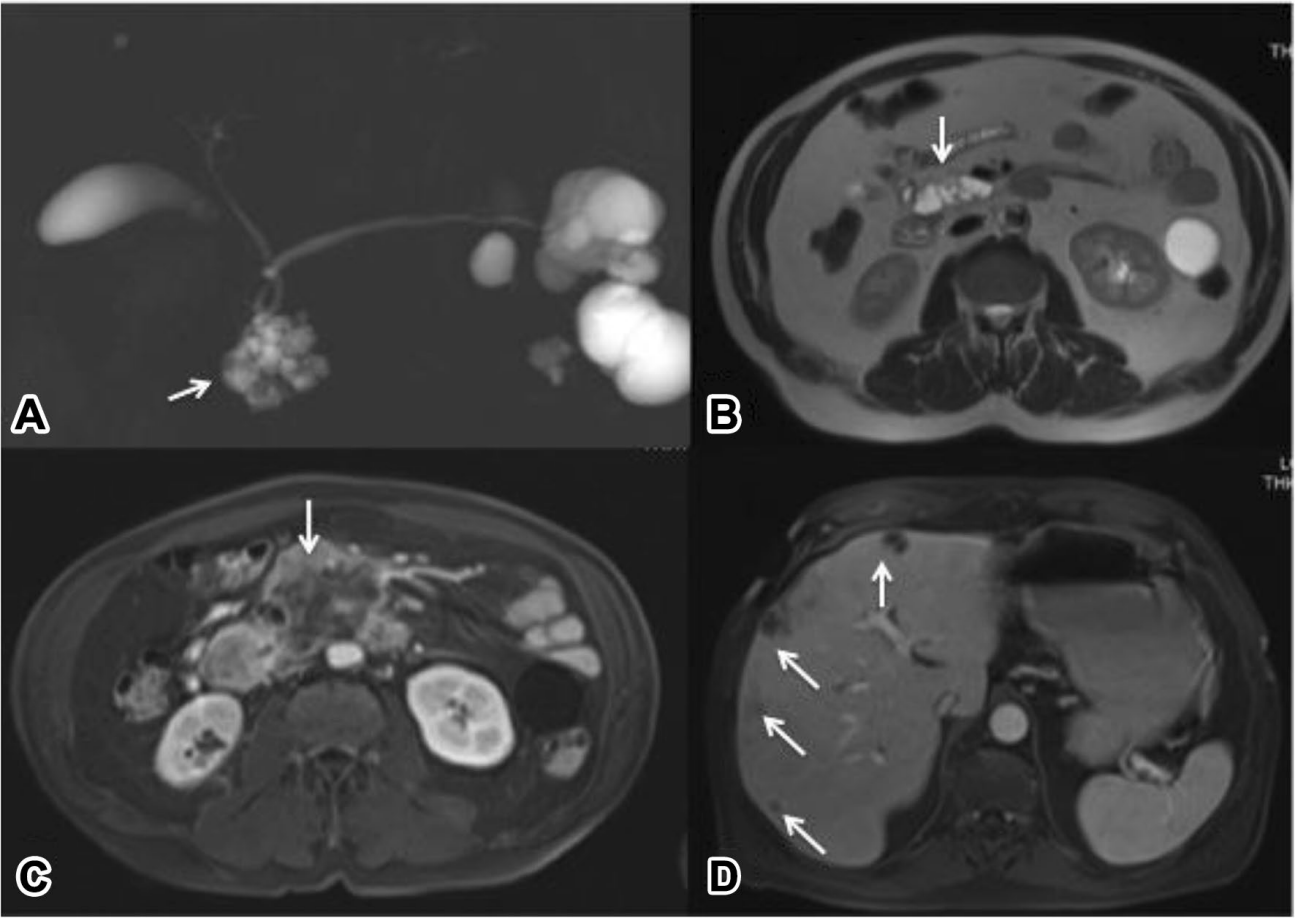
Degenerated IPMN. At MRCP a BD-IPMN is appreciable in the uncinate process. The lesion does not show solid nodules at T2 HASTE, as well as at DWI and contrast-enhanced MRI (not shown). Even after EUS no worrisome features were appreciated. At follow-up after 10 months a large hypovascular lesion occupies the uncinate process (arrow: C), with multiple hepatic metastases (arrows: D)
